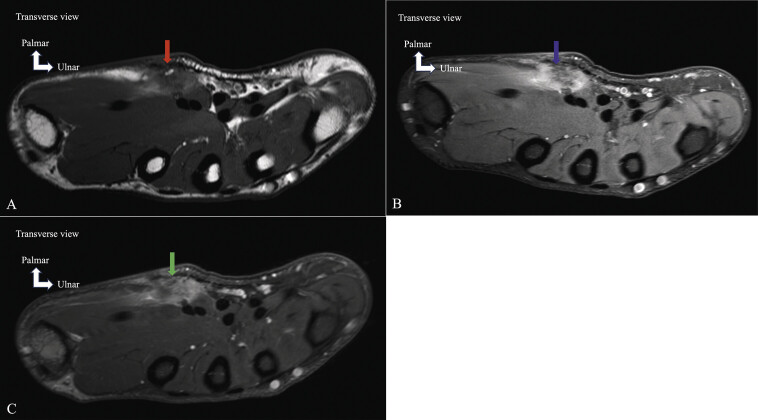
Epithelioid sarcoma of the thenar eminence. Axial T1-weighted image
